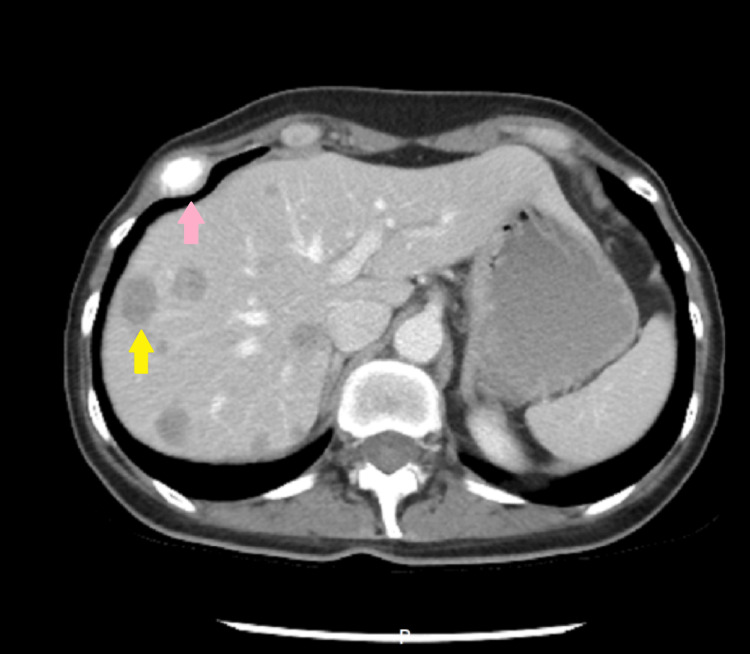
Abdominal CT-scan (spatial resolution: 512 × 512 and slice thickness: 5 mm). One of the multiple liver metastases with a yellow arrow and with pink arrow an osteolytic lesion on the seventh rib CT scan with intravenous contrast substance – axial image.
